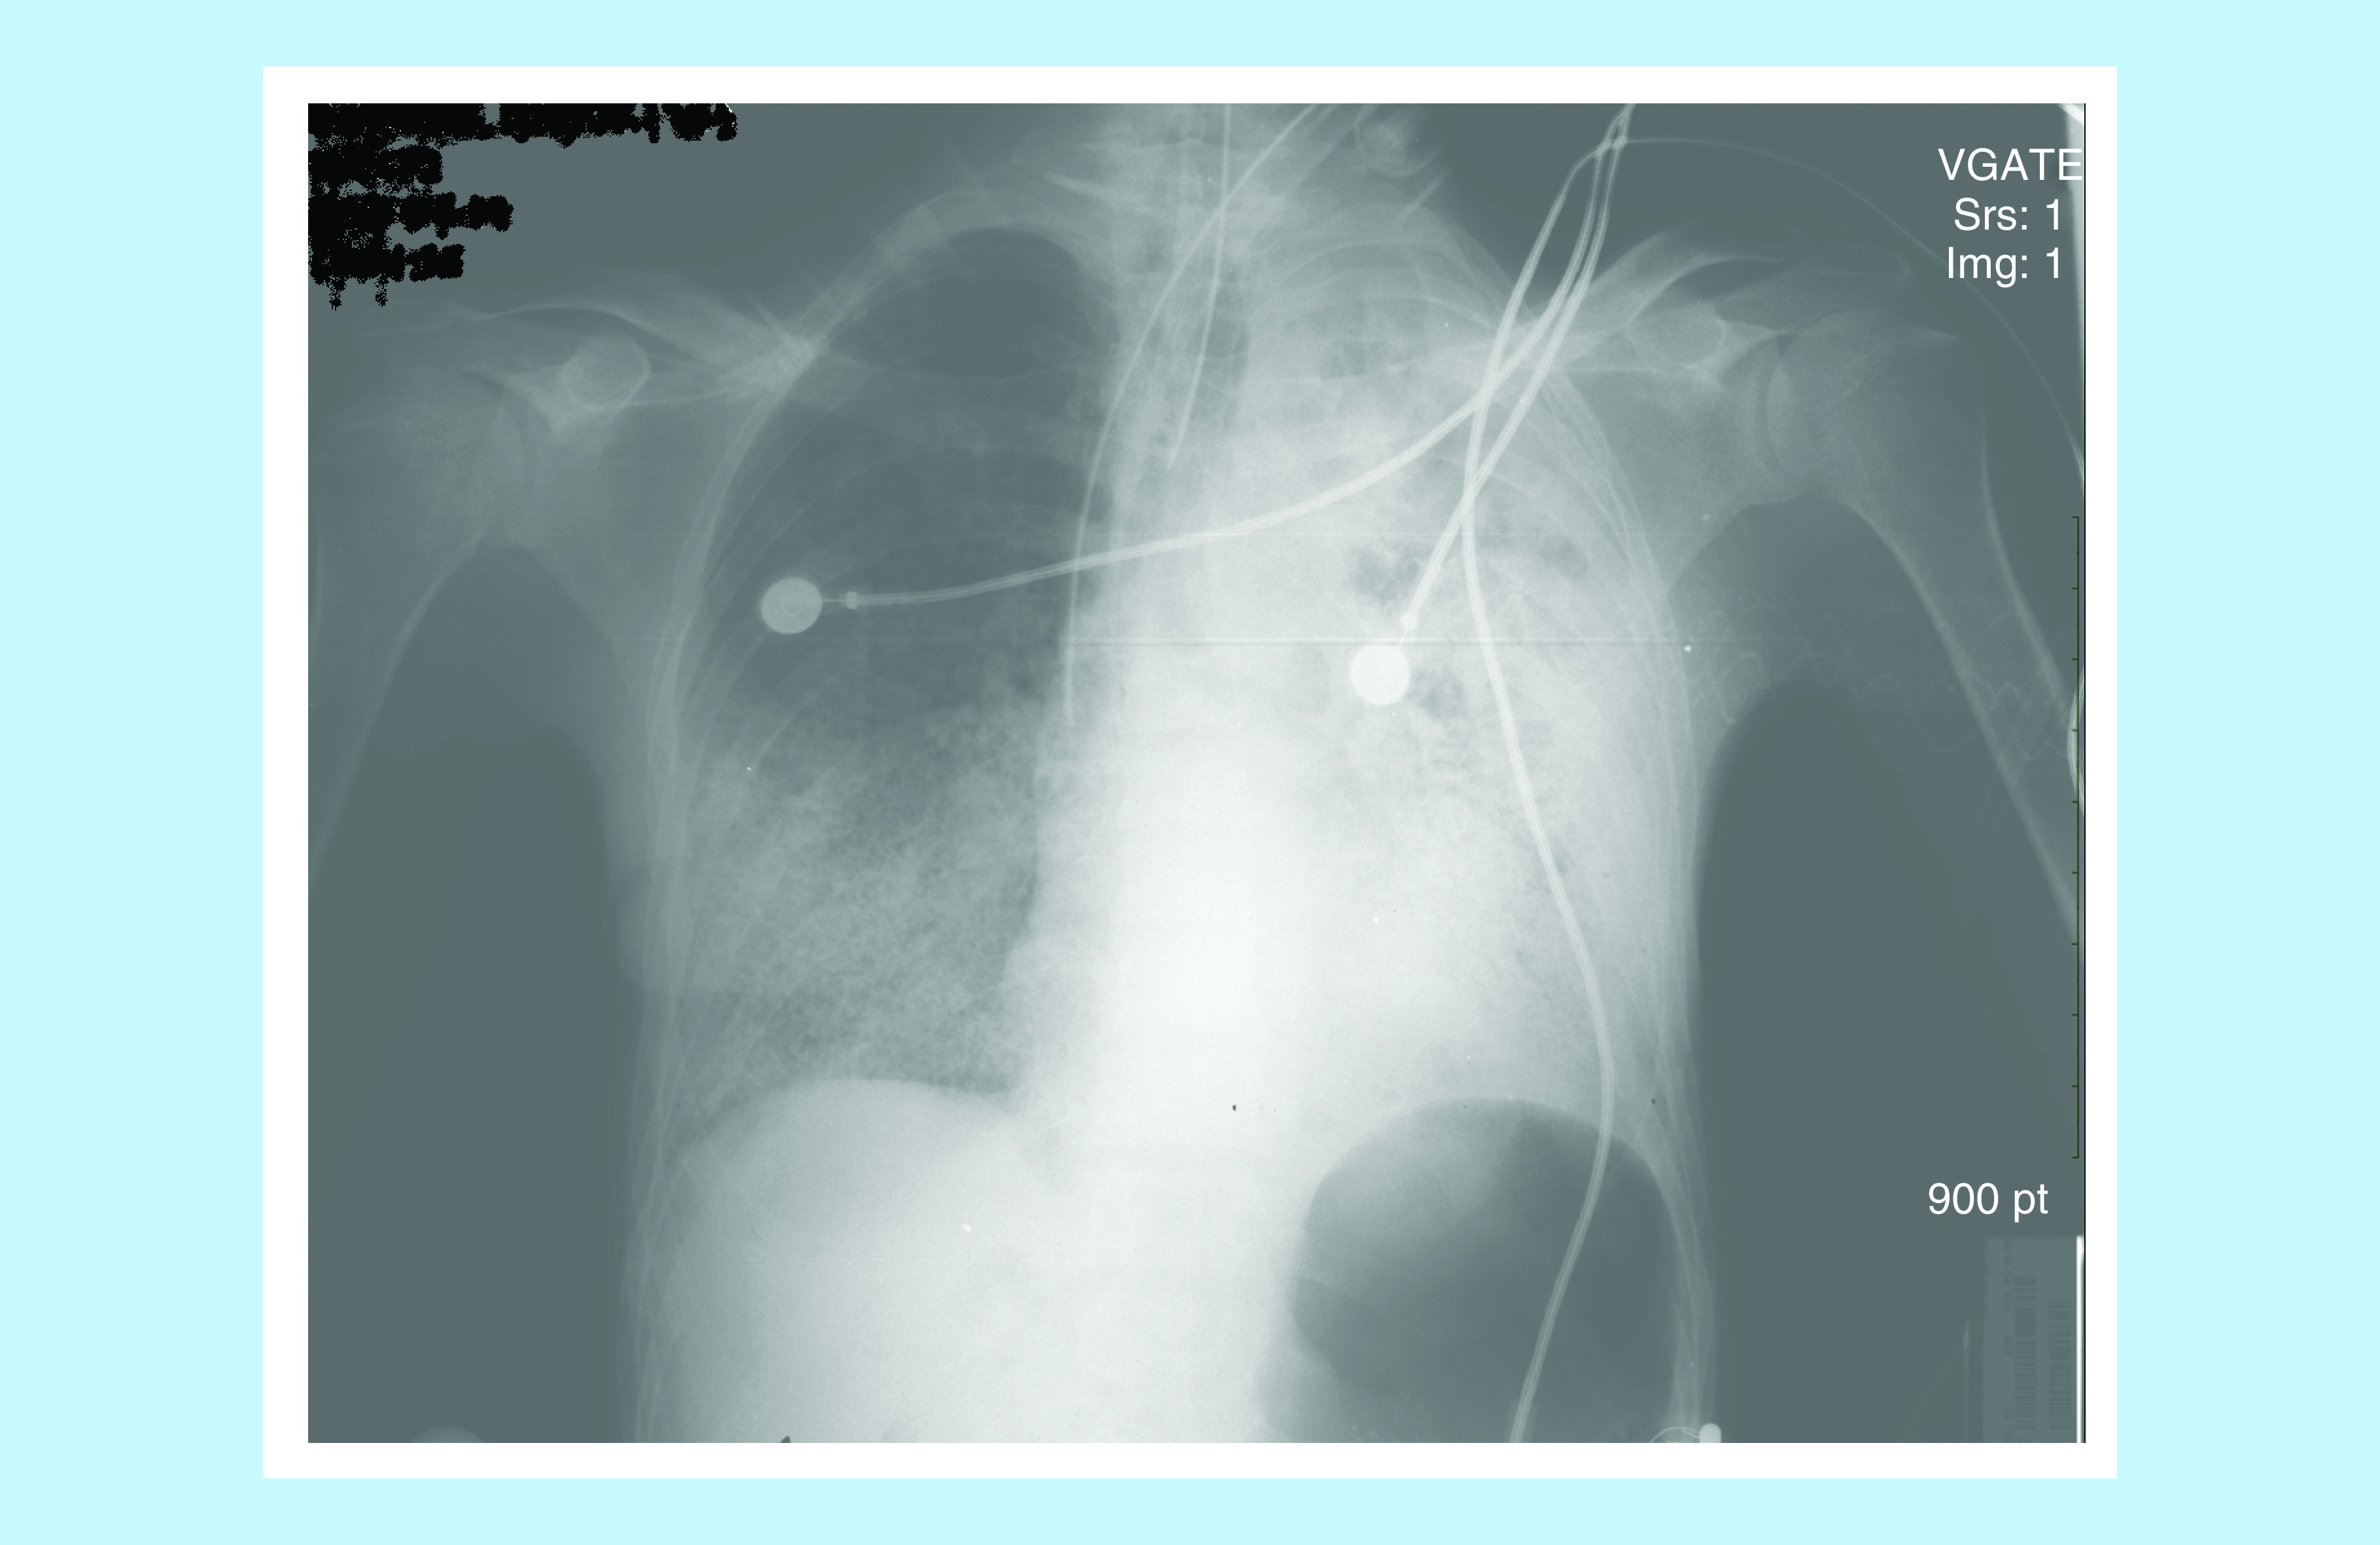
Chest x-ray of the patient with tuberculosis and acute respiratory distress syndrome.
